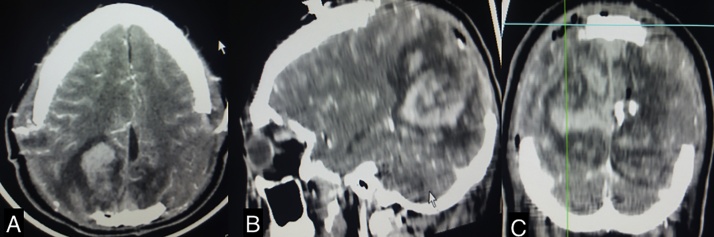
Axial, sagital and coronal postsurgical CT scan showing adequate cerebral decompression.
